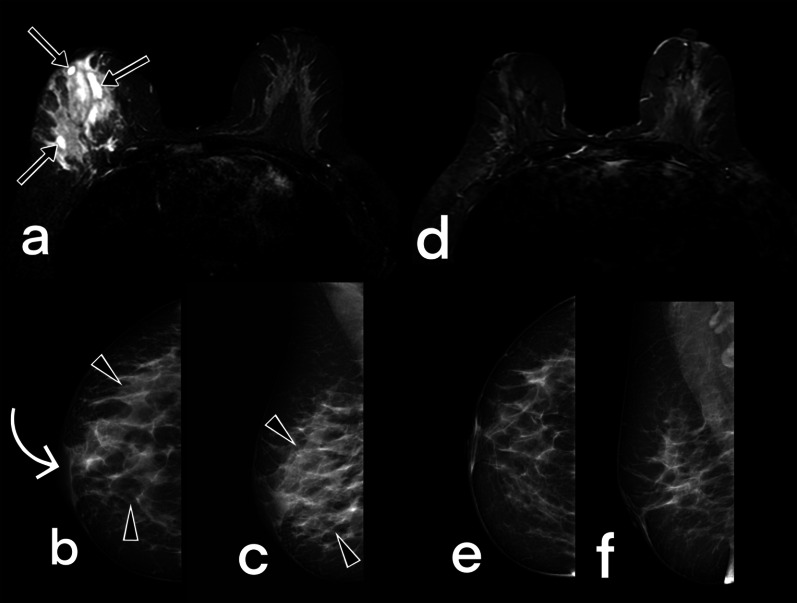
Images of a 44 years-old female patient obtained before (a–c) and 12 months after (d–f) antituberculous therapy. T2-weighted fat-suppressed axial image (a) demonstrates fluid collections (arrows) and a diffuse signal increase in the right breast. Mammograms (b, c) show a diffuse increase (BIRADS 4) in parenchymal opacity (arrowheads) and thickening of the skin (curved arrow). After the treatment, complete regression (BIRADS 1) of the findings is observed (d–f)
